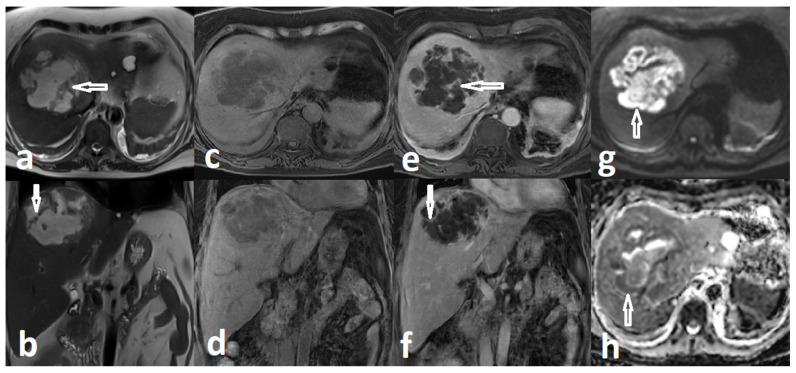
Hepatic abscess in MR scan, from left: T2WI in axial (a) and coronal (b) plane, T1WI without contrast in axial (c) and coronal (d) plane, T1WI with contrast in axial (e) and coronal (f) plane, DWI (g) and ADC map (h) in axial plane. Coronal images show abscess reaching the surface of the liver with abutment of the diaphragm. Abscess content demonstrates high T2W signal, low T1W signal and inhomogeneous restriction diffusion in DWI/ADC—predominantly high signal in DWI (short arrow in (g)) and predominantly low signal, especially peripherally, on ADC map (short arrow in (h)). T2W images clearly visualize low signal internal septa (short arrows in (a,b)). Post contrast T1W images show enhancement of irregular internal septa (short arrows in (e,f)).
